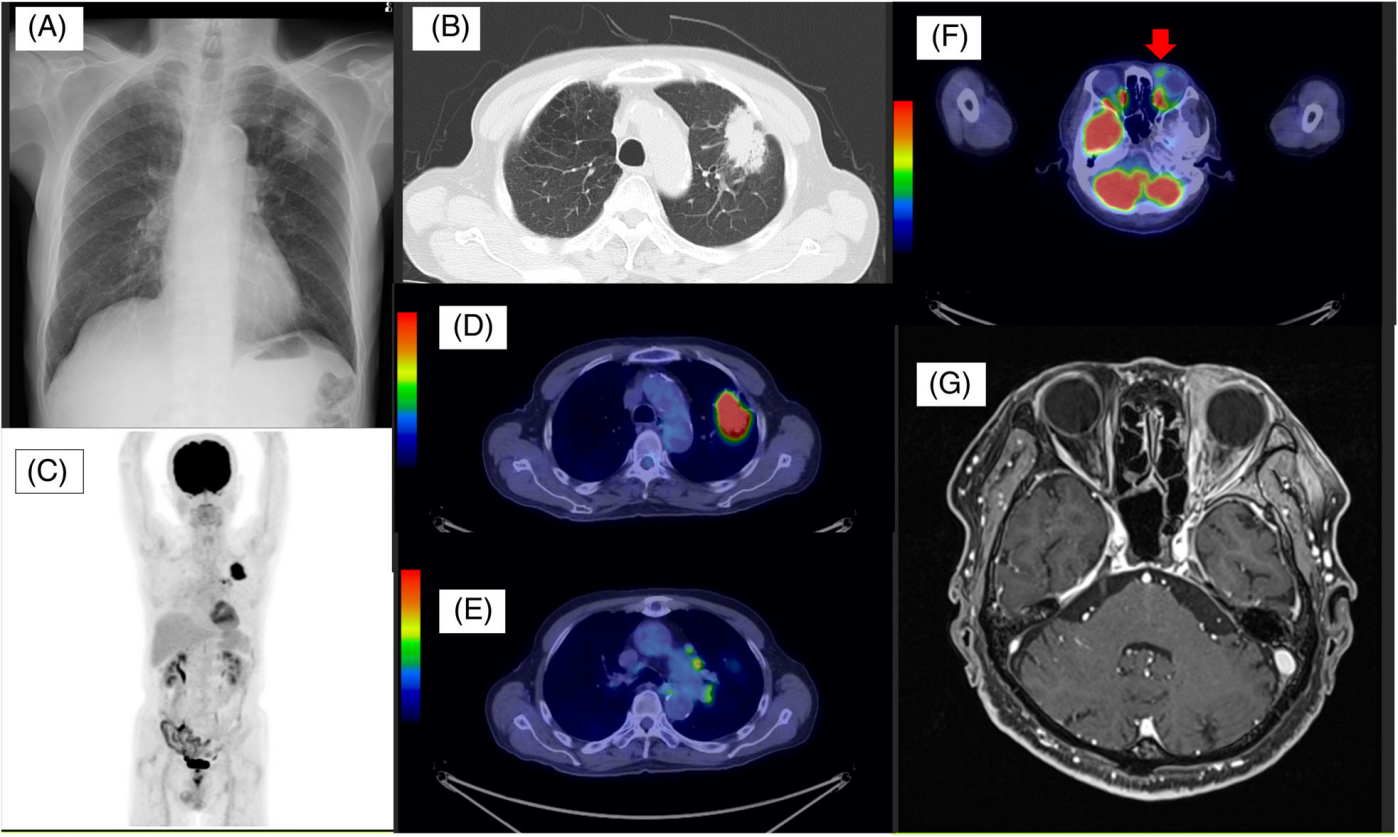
Radiographic findings on the diagnosis. (A) Chest radiograph at the initial visit to our hospital. (B) Primary lung cancer in the left upper lobe, revealed by computed tomography. (C) Overview of fluorodeoxyglucose F18 positron emission tomography (FDG PET). (D, E) FDG accumulation in the primary lung cancer and mediastinal lymph nodes on FDG PET. (F) Orbital mass on FDG PET. (G) Orbital mass on contrast‐enhanced MRI.
